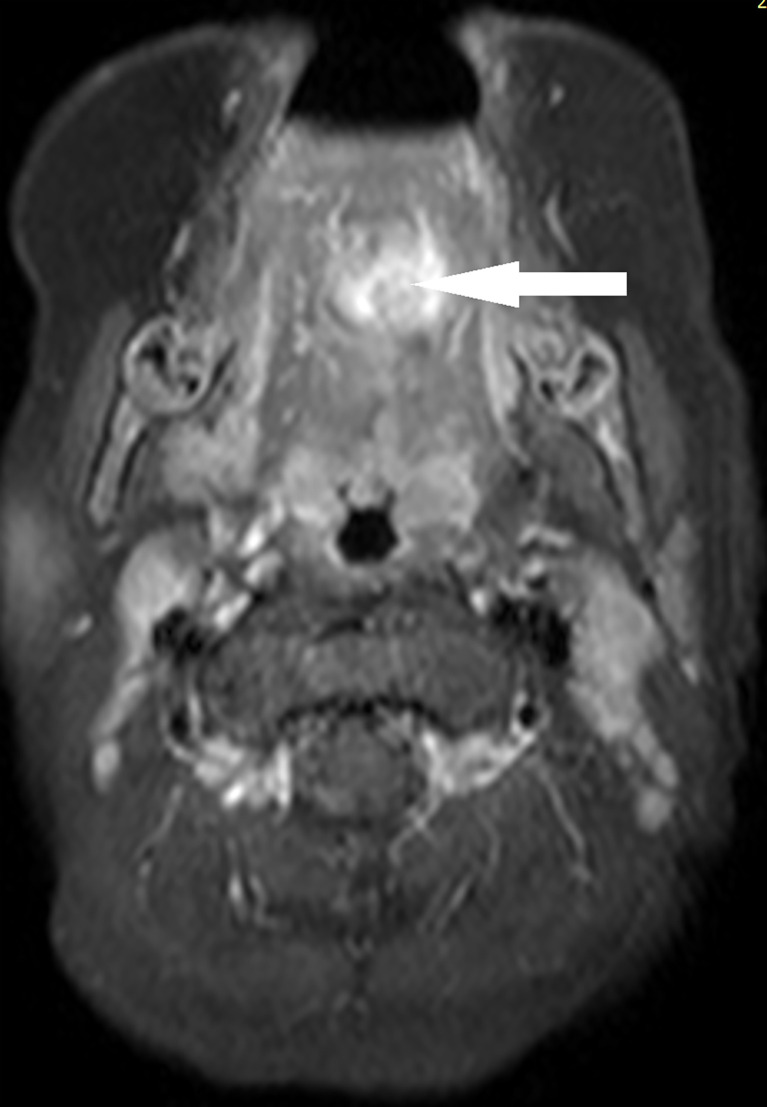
Case 2, tumor in magnetic resonance imaging at relapse (axial).
